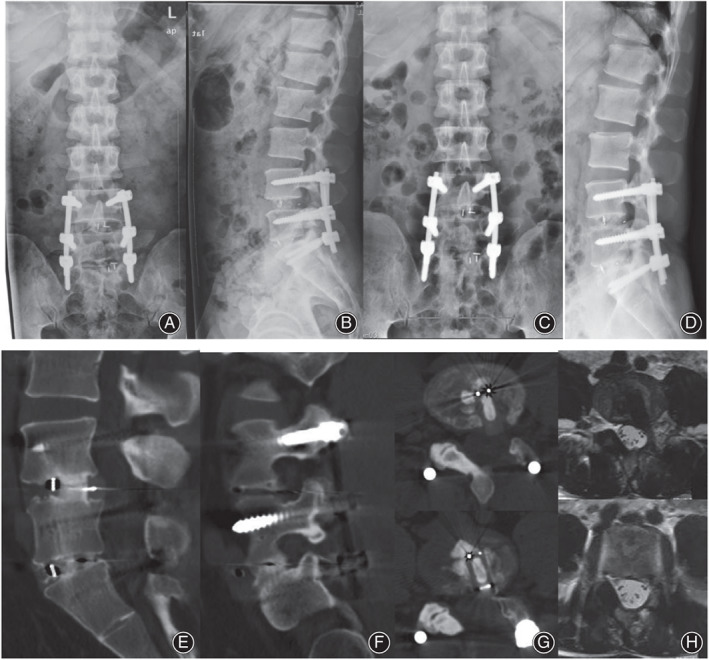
Imaging material of pre‐ and post‐operation. (A, B) Postoperative anterioposterior and lateral radiographs after L4/5 and L5/S1 TLIF in 2014; (C, D) X‐ray of lumbar spine in the patient on admission of our hospital in 2018; (E–G) CT of lumbar spine in the patient on admission of our hospital in 2018; (H) MRI of lumbar spine in the patient on admission of our hospital in 2018
